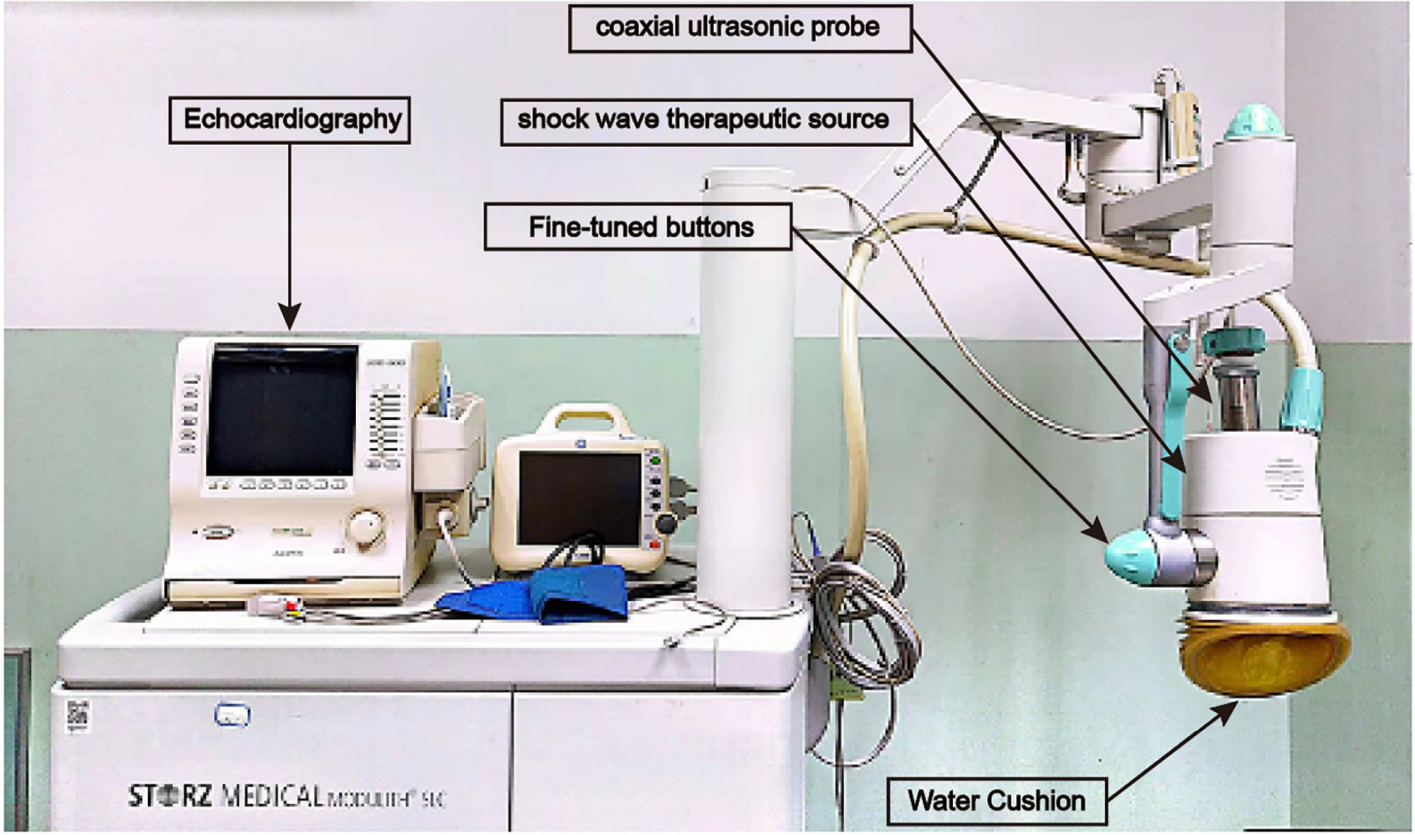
The machine is equipped with a shoch wave therapeutic source and coaxial ultrasonic probe. The SW generator is attached to the chest wall of the patient when used. The SW pulse is easily focused on the ischemic myocardium under the guidance of echocardiography. There is no need of sedatives or anesthesia. Republished from PLOS ONE under a CC BY license, with permission from Hongyan Cai, original copyright 2023.
